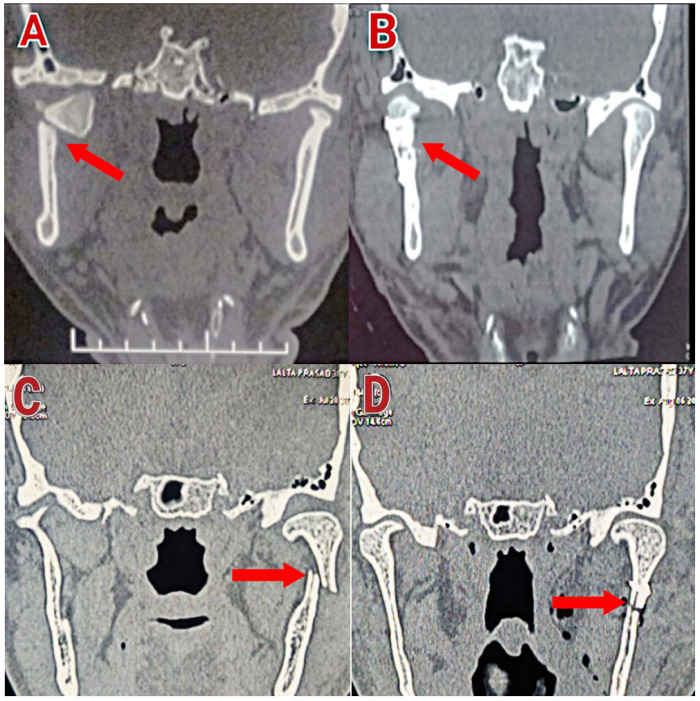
CT Image showing Pre-operative and Post-operative Reduction. (A) Pre-operative CT image of Condylar Base Fracture. (B) Post-operative CT image of Condylar Base Fracture. (C) Pre-operative CT image of Condylar Neck Fracture. (D) Post-operative CT image of Condylar Neck Fracture.
